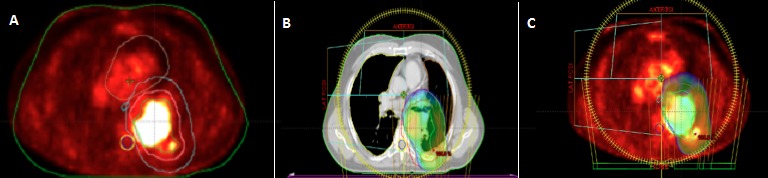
(A): target volume delineation in PET-CT; (B) et (C): coverage of the target volume by isodose line 95% (in blue)
